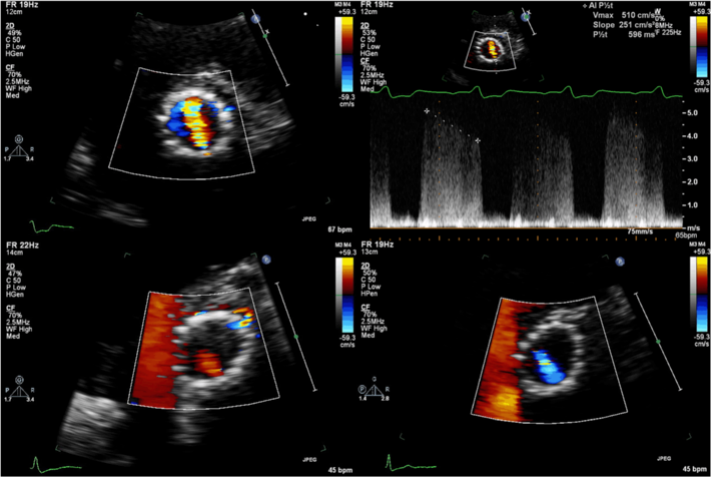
The origin of the PVL jet may be identified by Doppler imaging (*top images*) or the colour of the jet: red jet moving towards the transducer (*left, bottom image*) and blue jet moving away from the transducer (*right, bottom image*)
